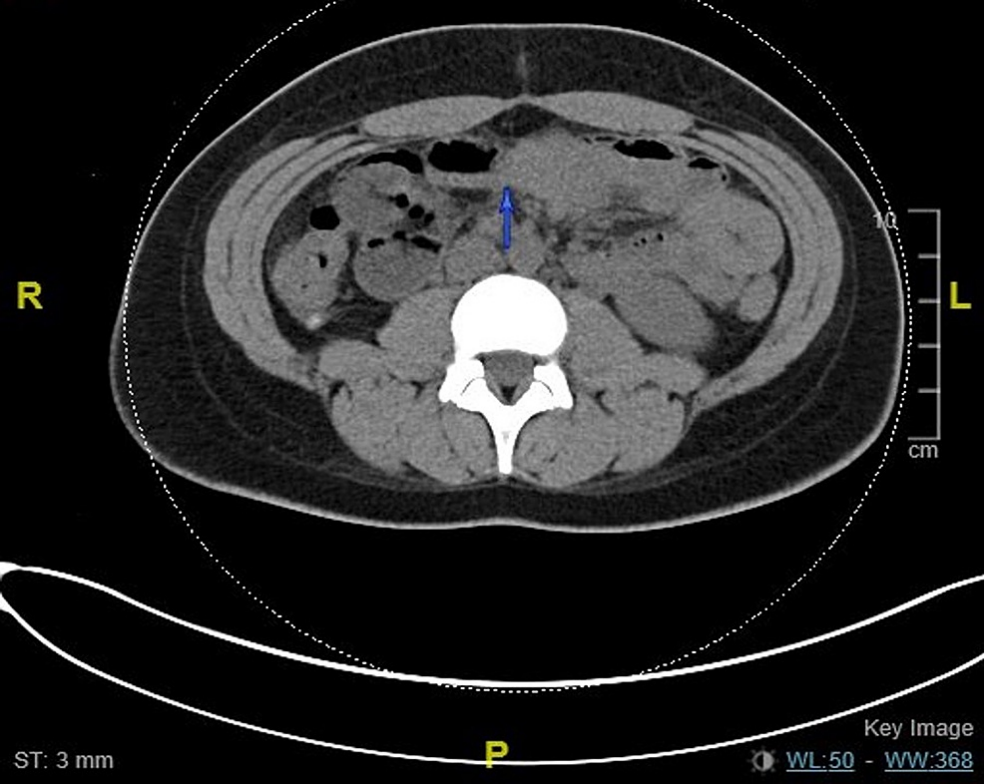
CT abdomen with the second transition point
